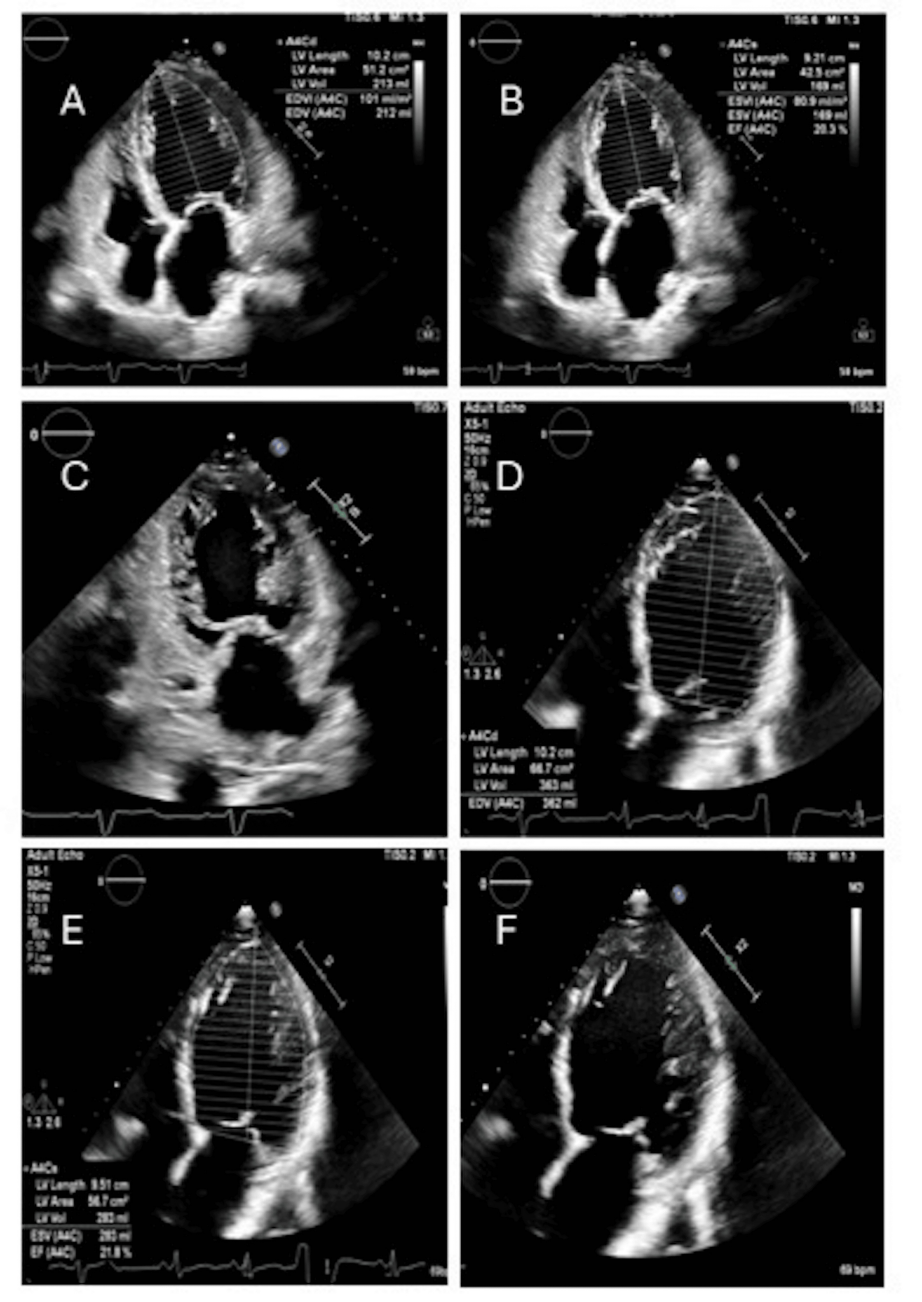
Transthoracic echocardiography (TTE) images for Case 1. (A-B) TTE with ejection fraction (EF) obtained by biplane/Simpson showing EF of 20.3%. (C) TTE showing trabeculations of the left ventricle (LV). (D-E) Repeat TTE showing EF of 21.8%, indicating no improvement. (F) TTE showing persistent trabeculations of the LV.
